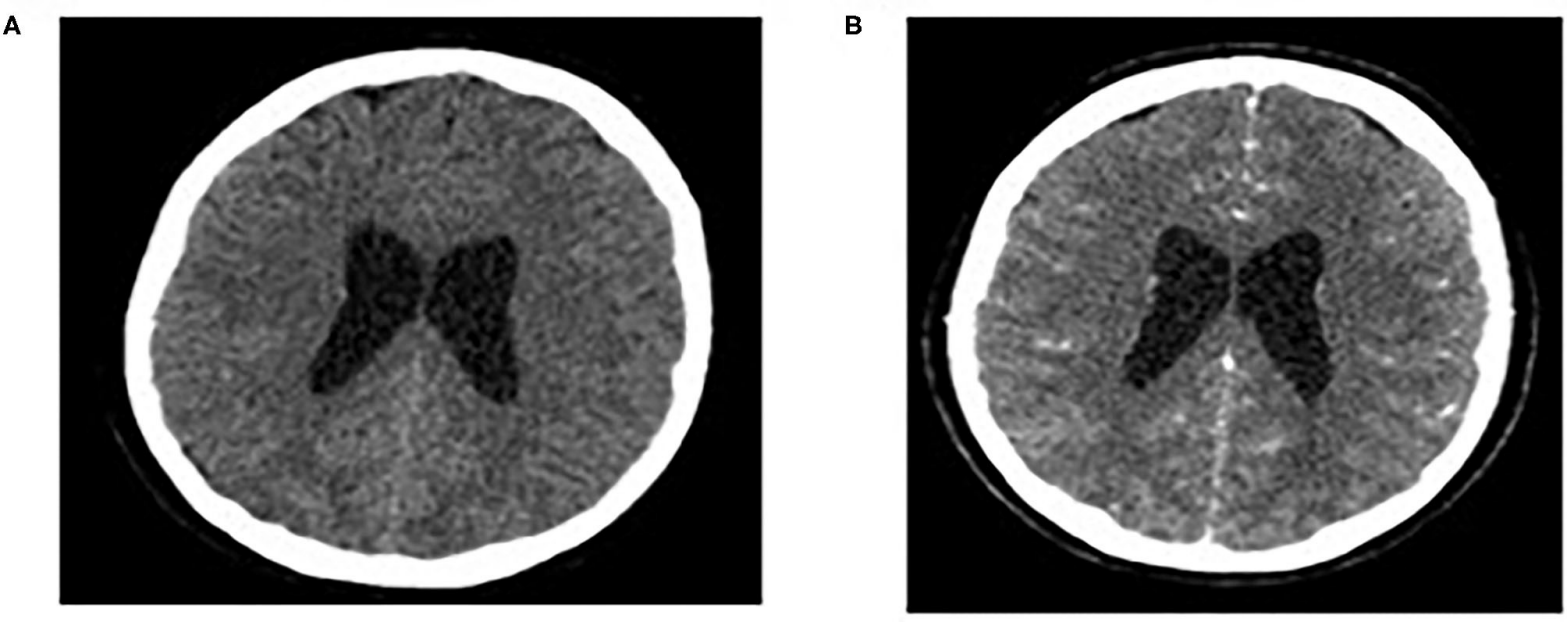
Normal findings of brain CT scan: CT brain scan without/with intravenous contrast dye administration (A,B).
